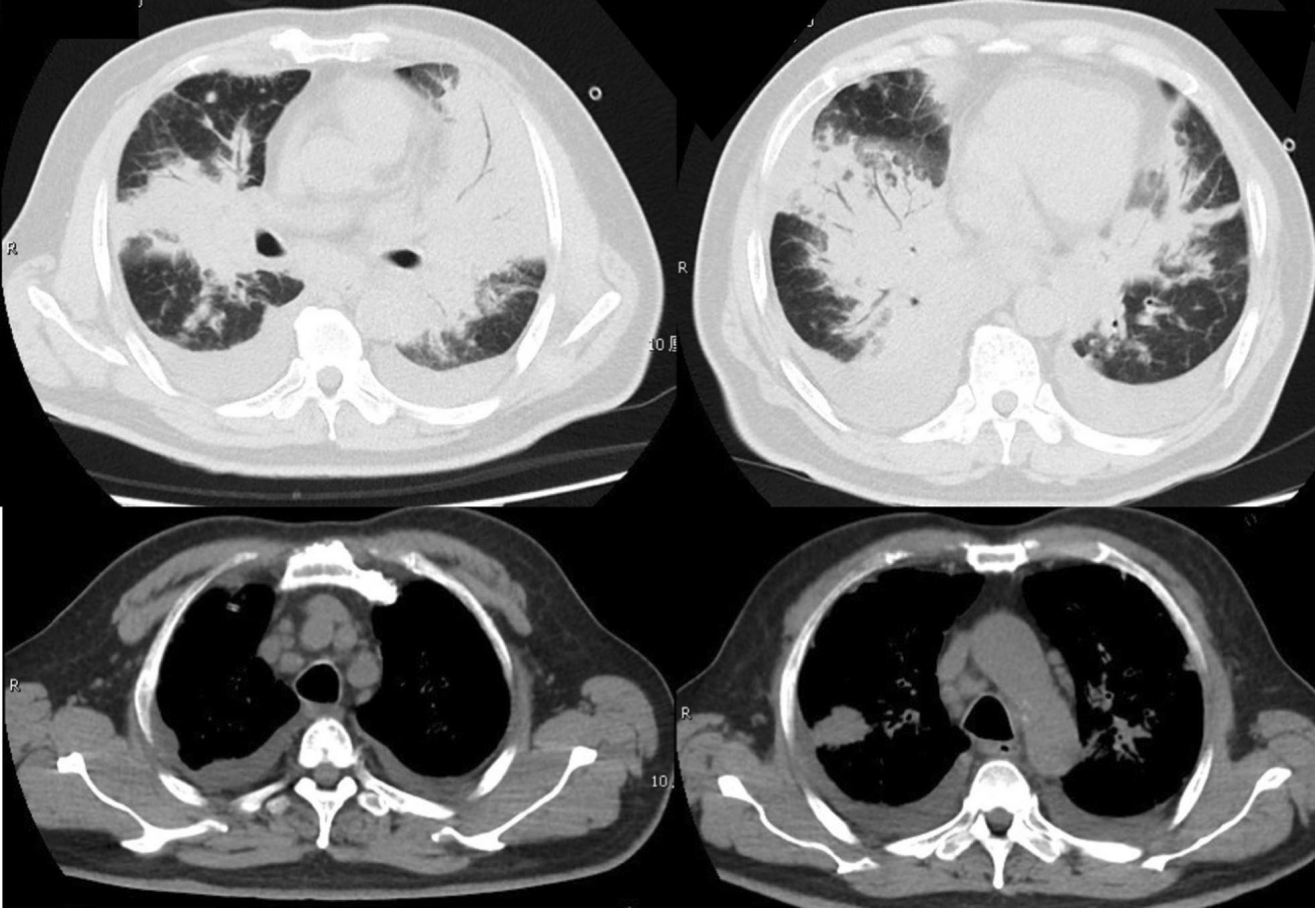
CT scan on day 10 after admission: both the consolidation and pleural effusion were exacerbated significantly
